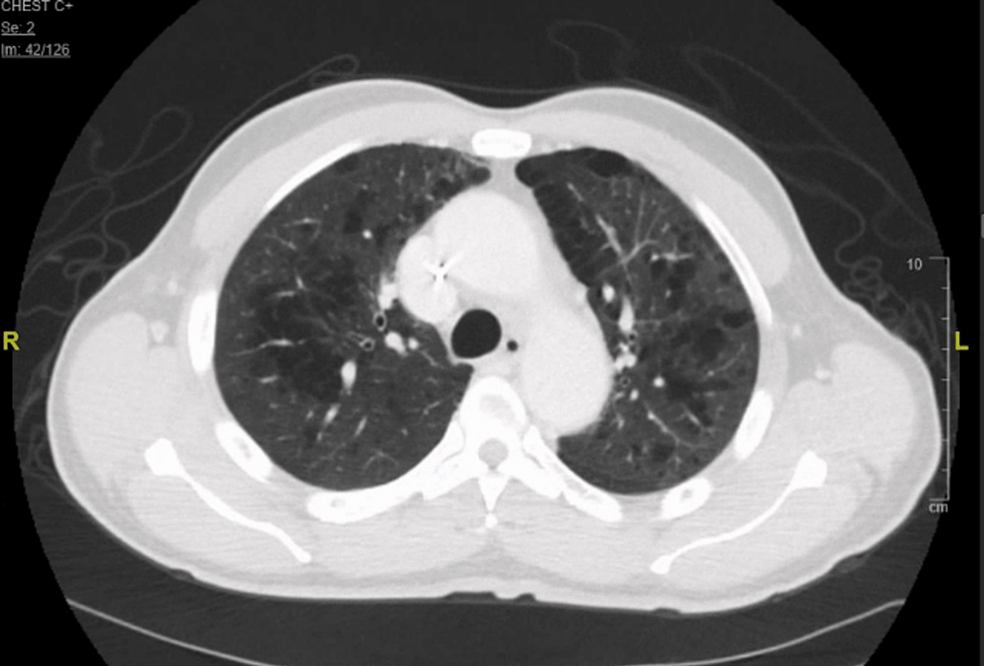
Initial CT chest prior to initiation of chemotherapy showing emphysematous changes
